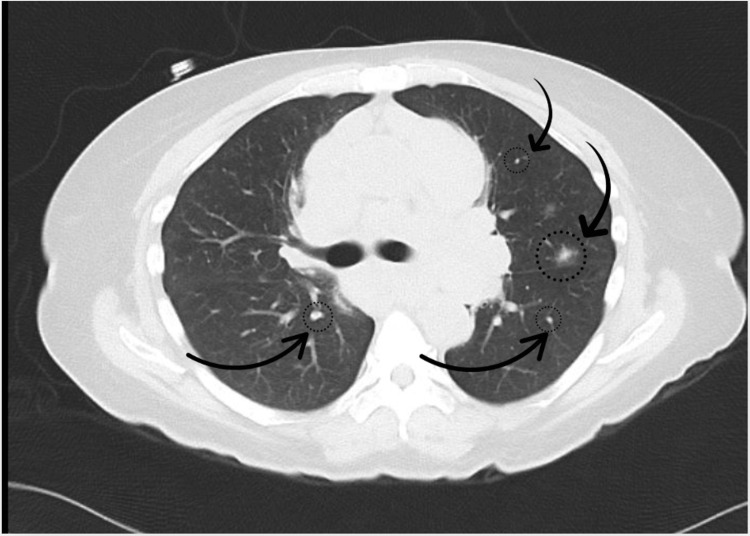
CT scan showing multiple pulmonary nodules (dotted circle with curved arrow) that may indicate metastatic disease CT: computed tomography
